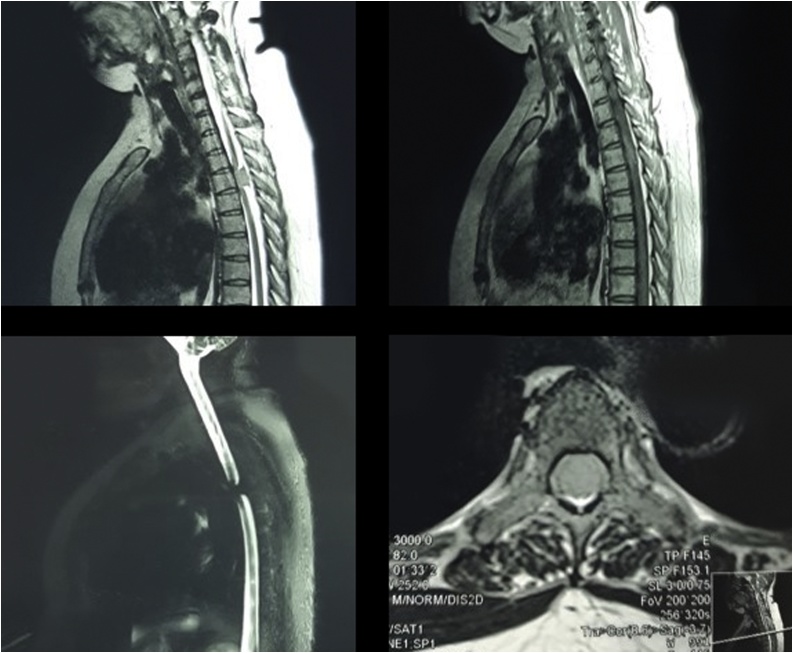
Magnetic Resonance Imaging revealed hypointense lesion extending from midbody of D3 to midbody of D4 vertebra.
